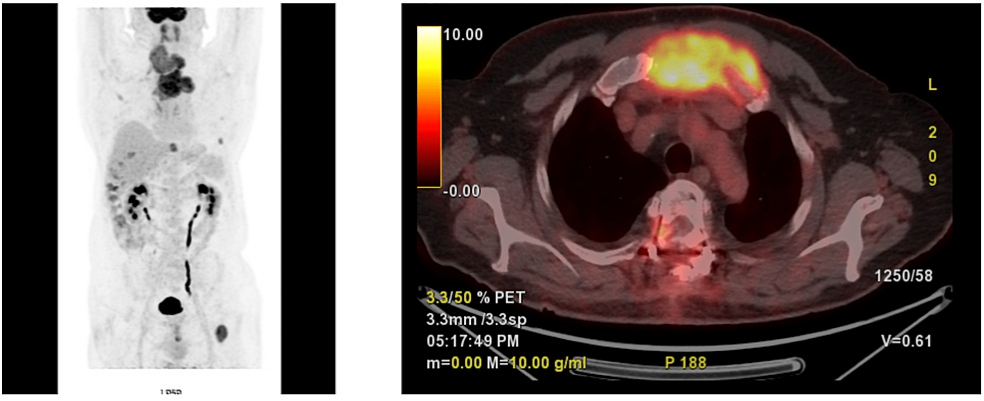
FDG-PET CT showed the sternal metastasis, which extended from the sternal notch to the third intercostal space. FDG: fluorodeoxyglucose, PET: positron emission tomography, CT: computed tomography.
